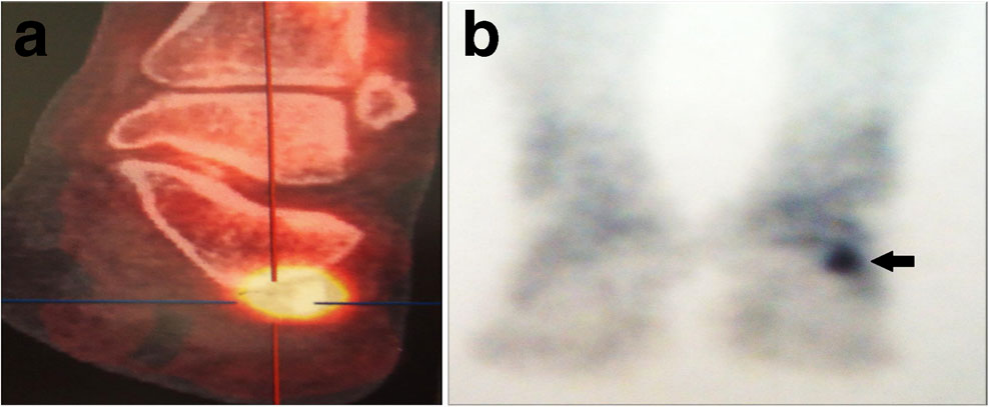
POPS – nuclear medicine. *10A* Positron emission tomography. *10B* Bone scintigraphy. Nuclear medicine can detect the uptake in the area of the OP (*arrow*) in chronic lateral foot pain
